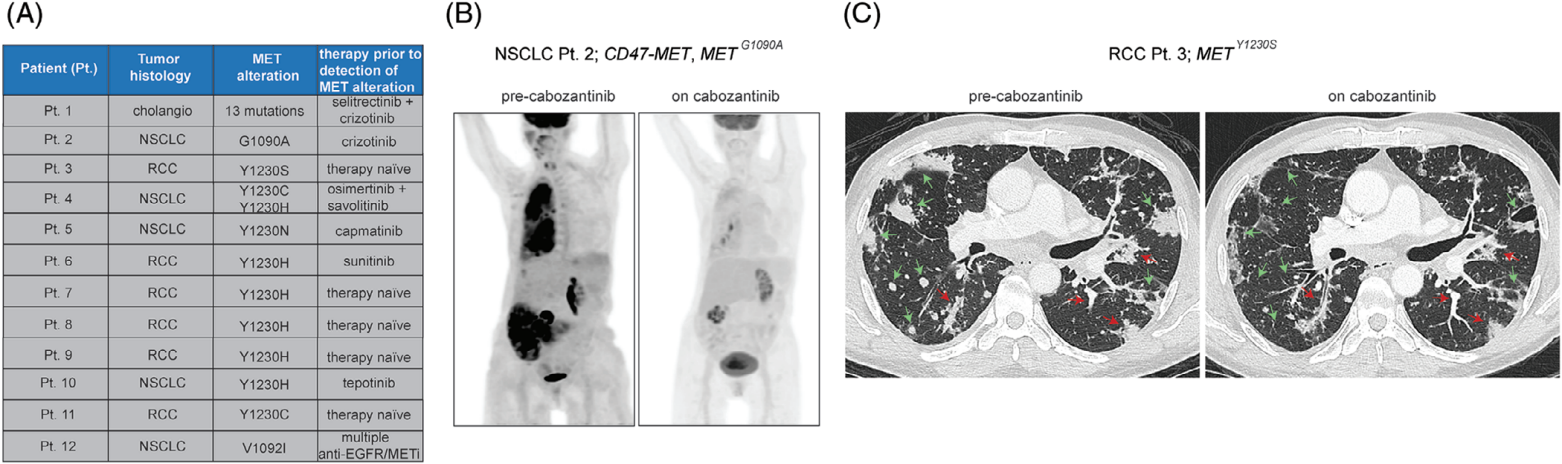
(A) List of patients identified whose tumours harbour MET mutations predicted to be sensitive to type II MET inhibitors. (B) PET scans showing the response of Pt. 2 (CD47‐MET, MET^G1090A^ mutant non‐small‐cell lung cancer [NSCLC]) to cabozantinib. (C) Computed tomography (CT) scans showing the response of Pt. 3 (MET^Y1230S^ mutant renal cell carcinoma [RCC]) to cabozantinib.
